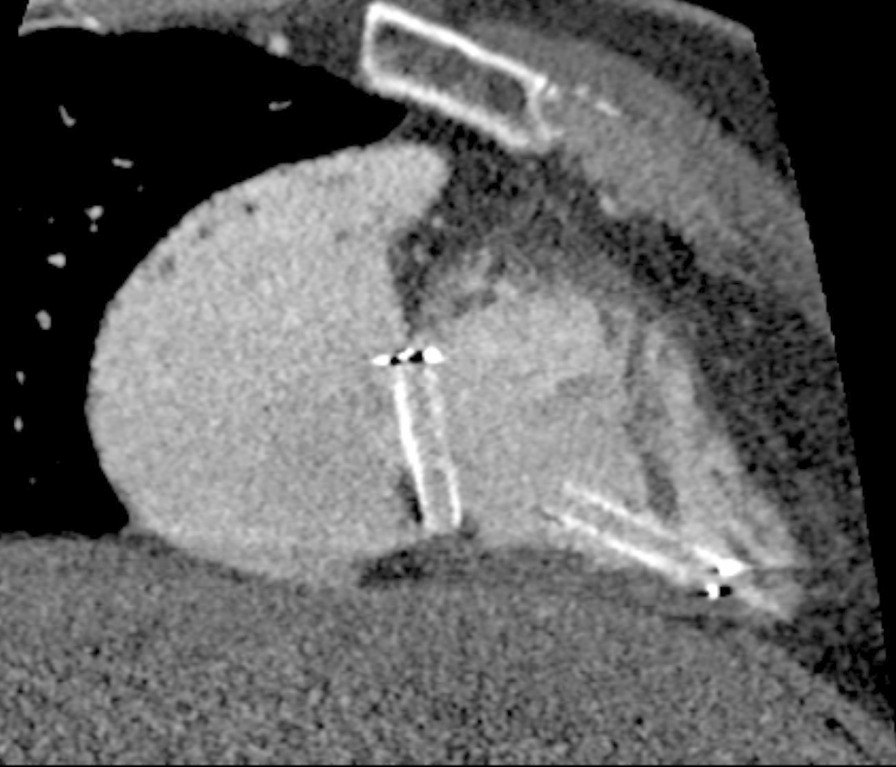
Cardiac CTA shows tubular high-density shadows at the tricuspid valve and in the right ventricle
